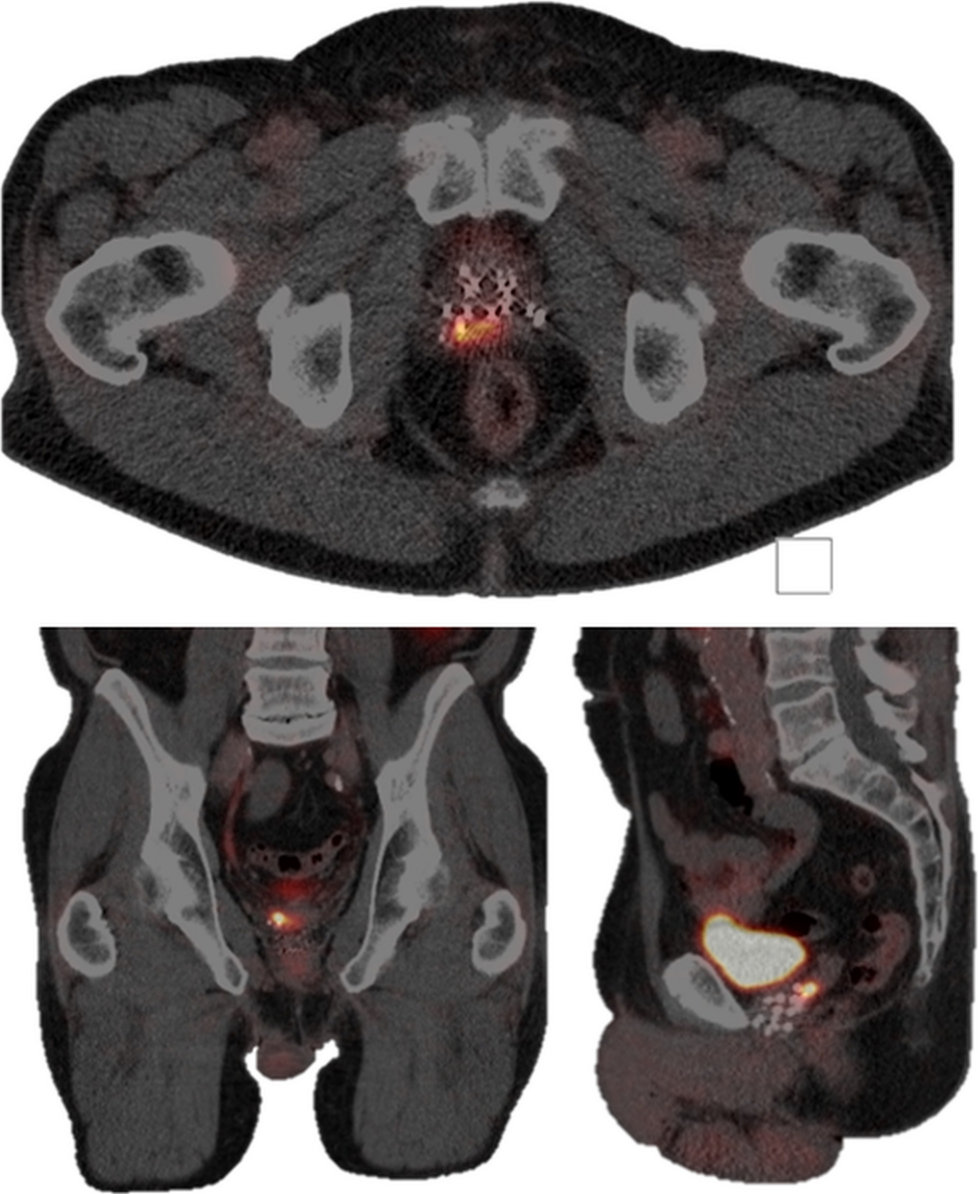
First recurrence lesion on 68Ga-PSMA PET-CT Transversal plane (upper image), coronal plane (lower-left image), and sagittal plane (lower-right image) showing the first recurrence lesion in the right dorsal peripheral zone and in the right seminal vesicle on the 68Ga-PSMA PET-CT, nine years after initial prostate cancer treatment.
